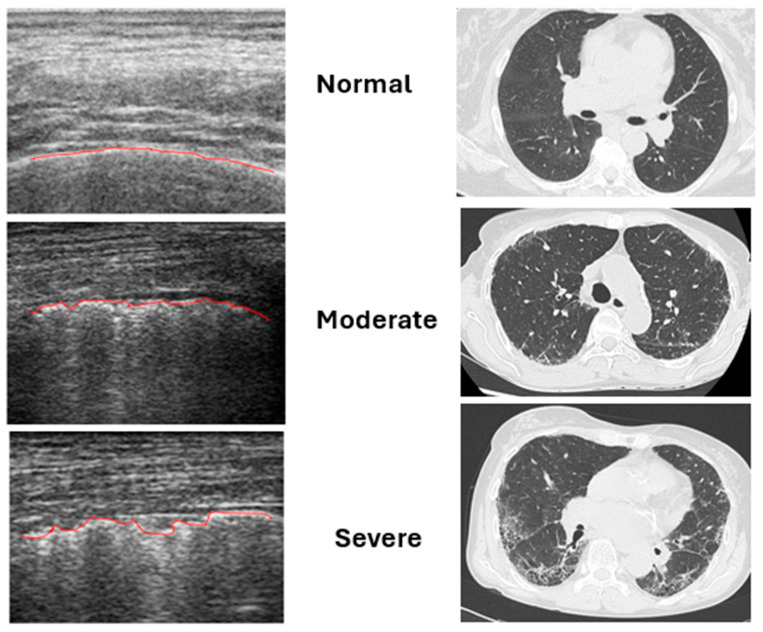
Correspondence between the severity of PIs and HRCT patterns. The red line represents the pleural line; its irregularities worsen in proportion to the severity of interstitial lung involvement.
